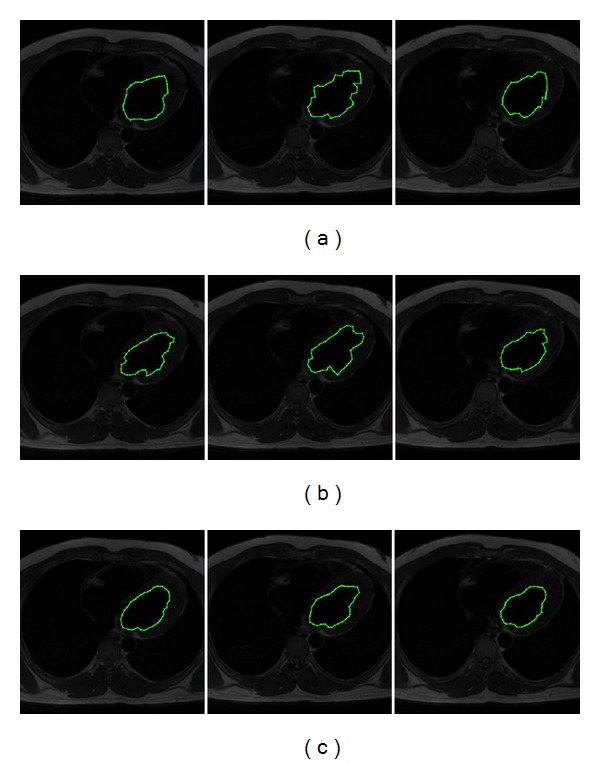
MR images (human left ventricle segmentation): (a) results of classical ACM, (b) results of Tseng method, and (c) results of MACDE implementation.
